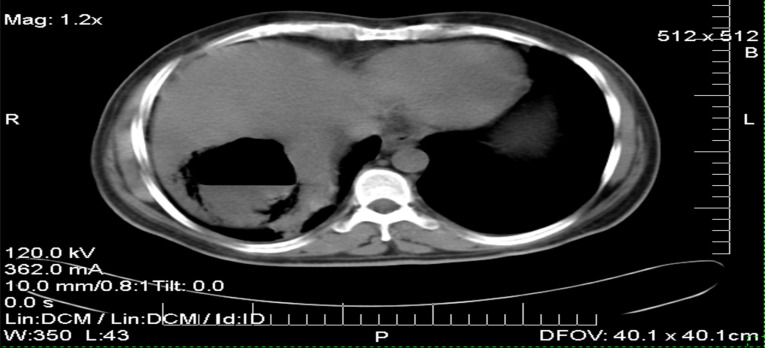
Tri-phasic abdominal CT scan on the initial presentation
